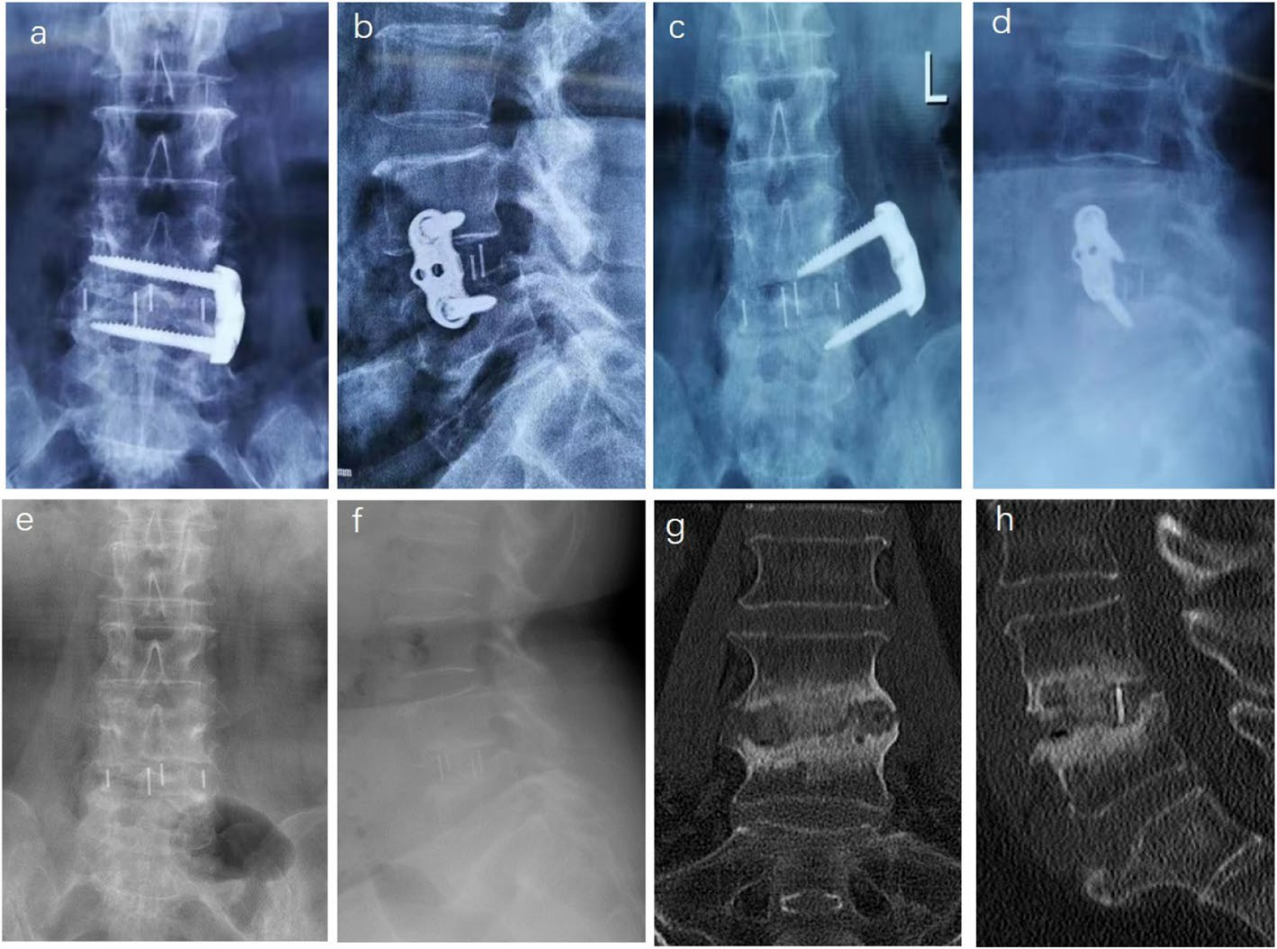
A case of failure of internal fixation. a, b Anterior and lateral X-ray after XLIF. c, d Anterior and lateral X-ray one month after operation. e, f Anterior and lateral X-ray after revision. g, h Coronal and sagittal CT one year after revision
